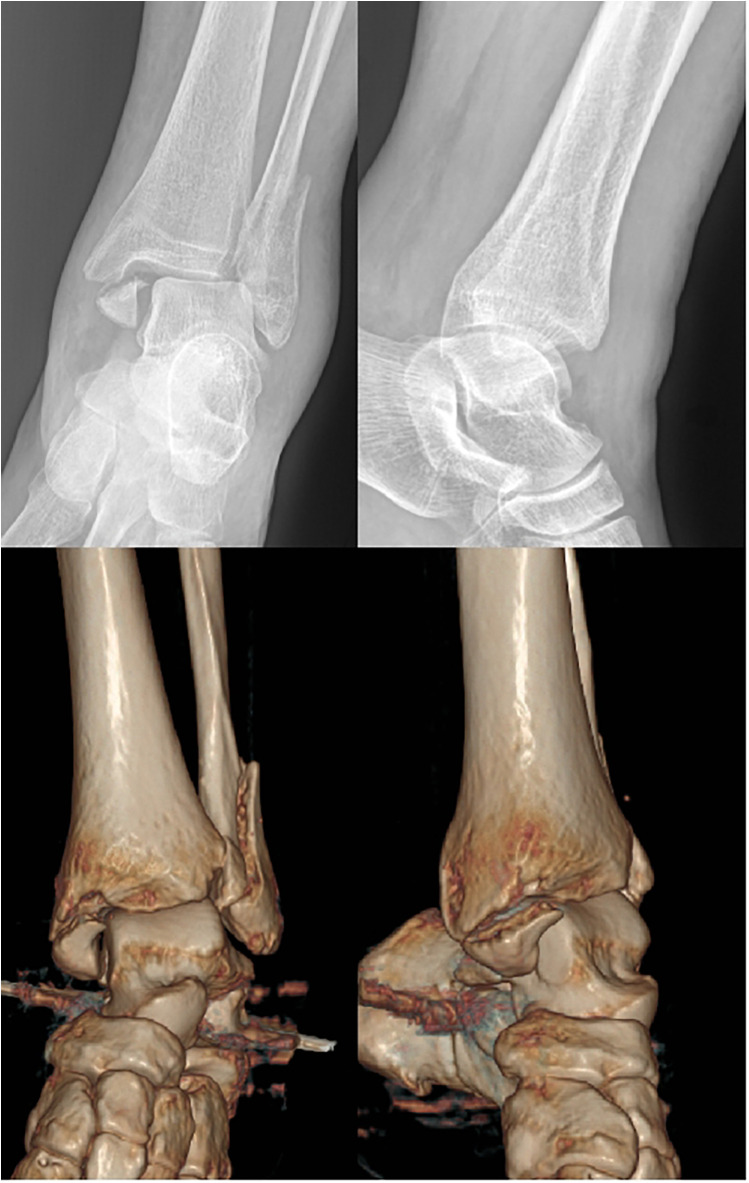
A 46-year-old female patient was admitted to the hospital due to “an accidental fall 3 h prior”. Imaging examinations of the affected limb, consisting of x-ray (upper two images) and 3D CT scan (lower two images) revealed fractures of the left medial and lateral malleoli accompanied by subluxation of the ankle joint.
